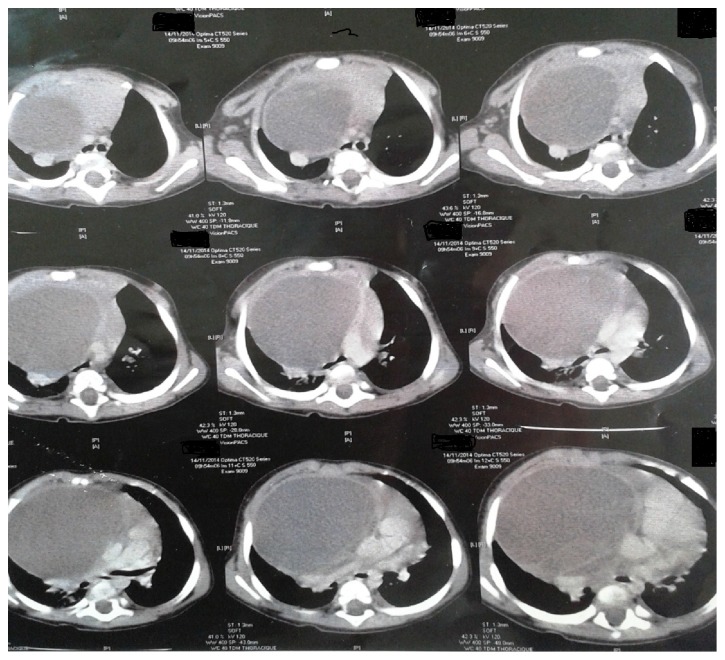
CT scan showing aspects of mediastinal teratoma: a combined cystic-solid tumour with walls of variable thickness.
